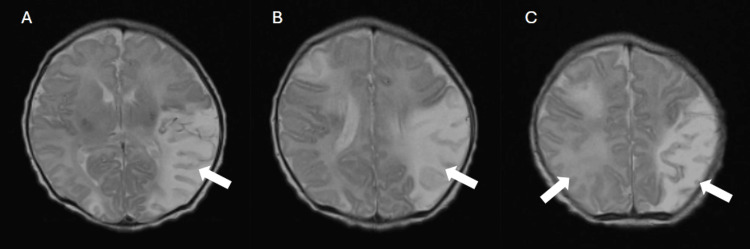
MRI of the head showing areas of infarction within the brain, demonstrated by the white arrows. Images A and B show areas of left middle cerebral artery infarction. Image C demonstrates bilateral middle cerebral artery infarctions, with the left side more affected than the right. These MRI findings place the baby at high risk for a later right-sided motor difficulty. She is also at risk of later mild cognitive and behavioural difficulties and may have an increased risk for later seizures.
